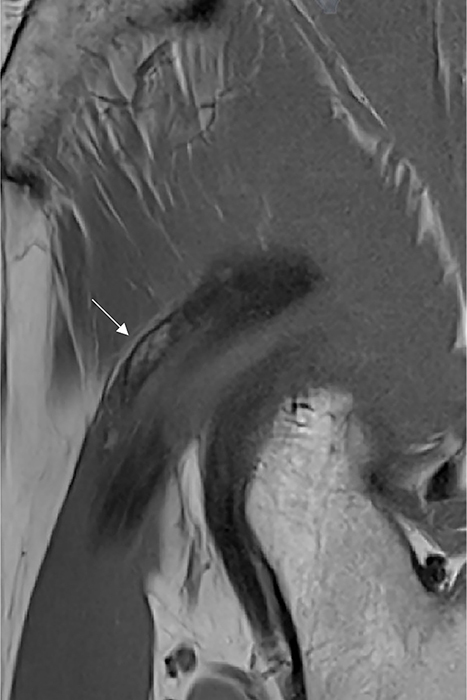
Magnetic Resonance Imaging shows mature HO containing fatty bone marrow along the course of the indirect head of the rectus femoris (arrow) on sagittal T1‑weighted images.
